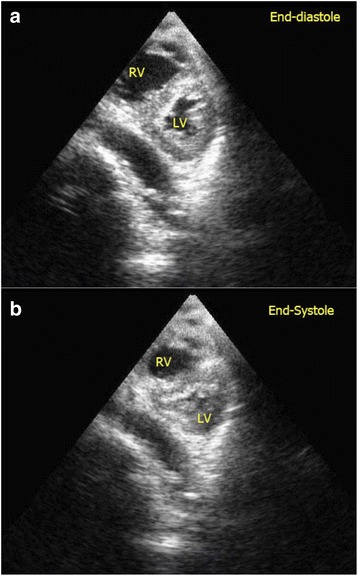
a, b Severe hypovolemic shock. Kissing walls of left ventricle on parasternal short axis view is shown. The left ventricle is obliterated during systole. This patient suffered from severe hypovolemia due to gastrointestinal bleeding. (*RV* right ventricle, *LV* left ventricle)
